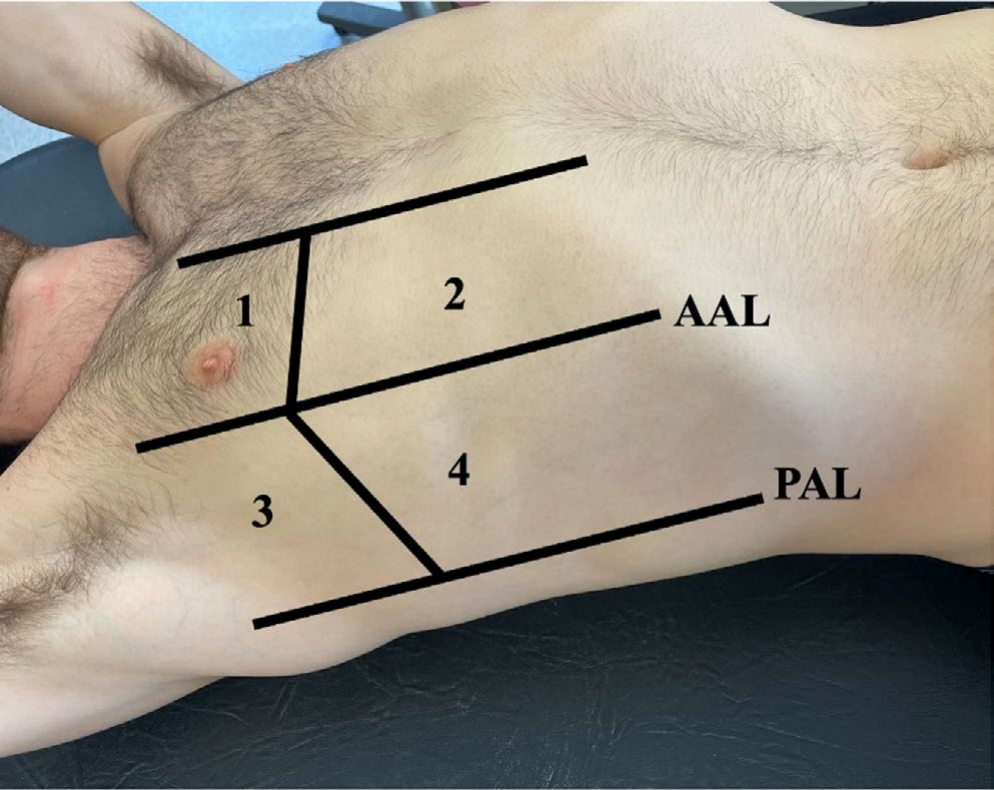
8‐Zone lung ultrasound examination of a representative participant. Areas 1 and 2 denote the anterior chest regions. Areas 3 and 4 denote the lateral chest regions. The regions were the same on the left side of the chest region as seen above (Volpicelli et al., 2006).
